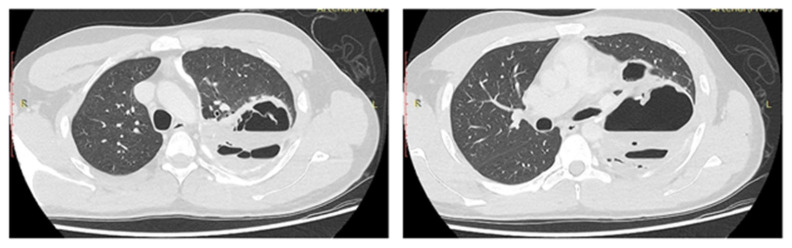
Computed tomography with intravenous contrast during the third postoperative week after redo left thoracotomy and lower lobectomy. The imaging was ordered to investigate fever and leukocytosis. Trapped lung (upper lobe) with air–fluid level and septae confirmed postoperative complex pleural empyema. This type of empyema is unlikely to respond to percutaneous drainage and requires revision surgery for debridement, decortication, and possibly thoracoplastic procedures (e.g., Eloesser flap).
